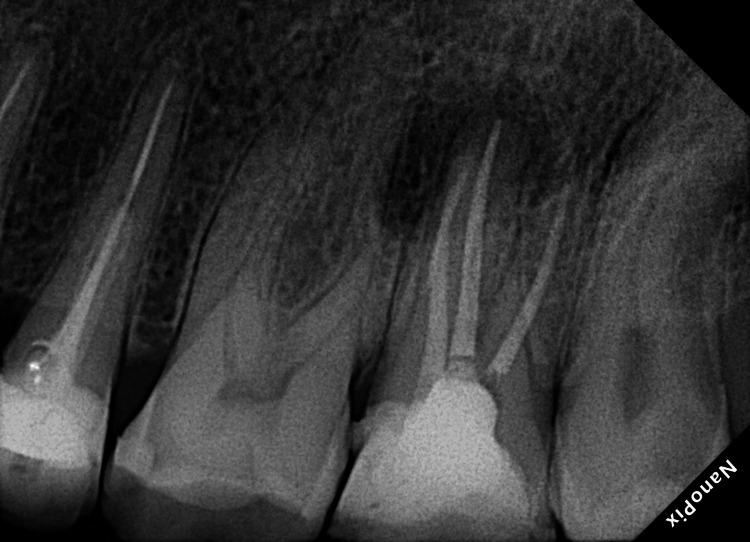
One-year follow-up radiograph showing healing of the periapical lesions
